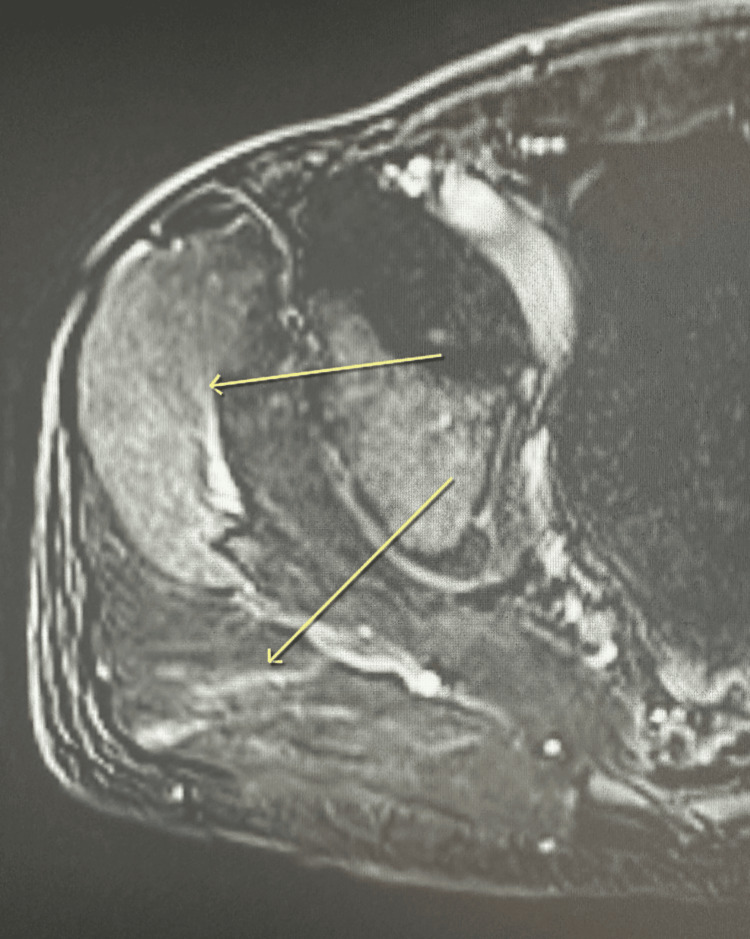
Axial pelvic MRI (postcontrast T1 fat-saturated), demonstrating multifocal intramuscular enhancement in gluteus maximus and minimus (see yellow arrows) MRI: magnetic resonance imaging
